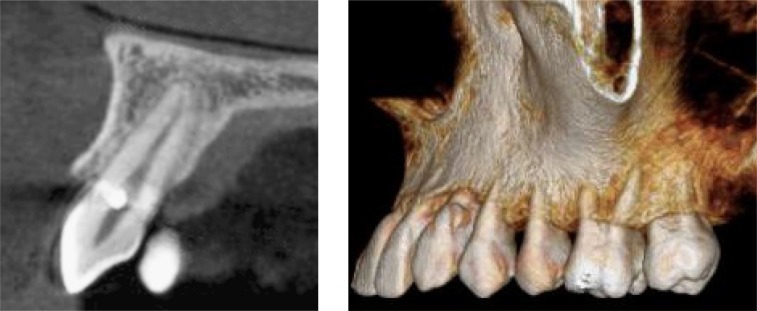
Sagittal tomographic slice and 3D CT scan of External Cervical Resorption
